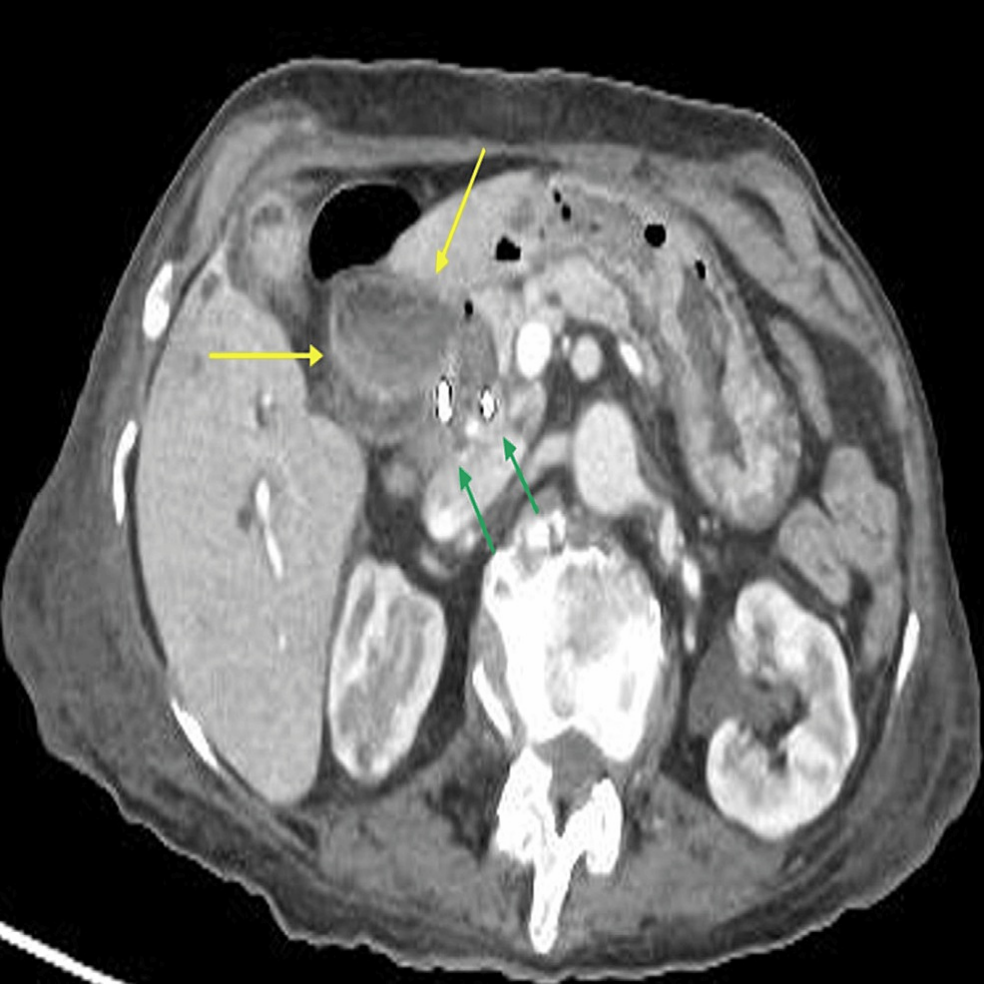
Abdominal CT axial image with high attenuated blood products along the intrahepatic and dilated biliary ducts (yellow arrows). Also seen are pigtail stents inserted previously during ERCP to help drainage (green arrows). CT: computed tomography; ERCP: endoscopic retrograde cholangiopancreatography
